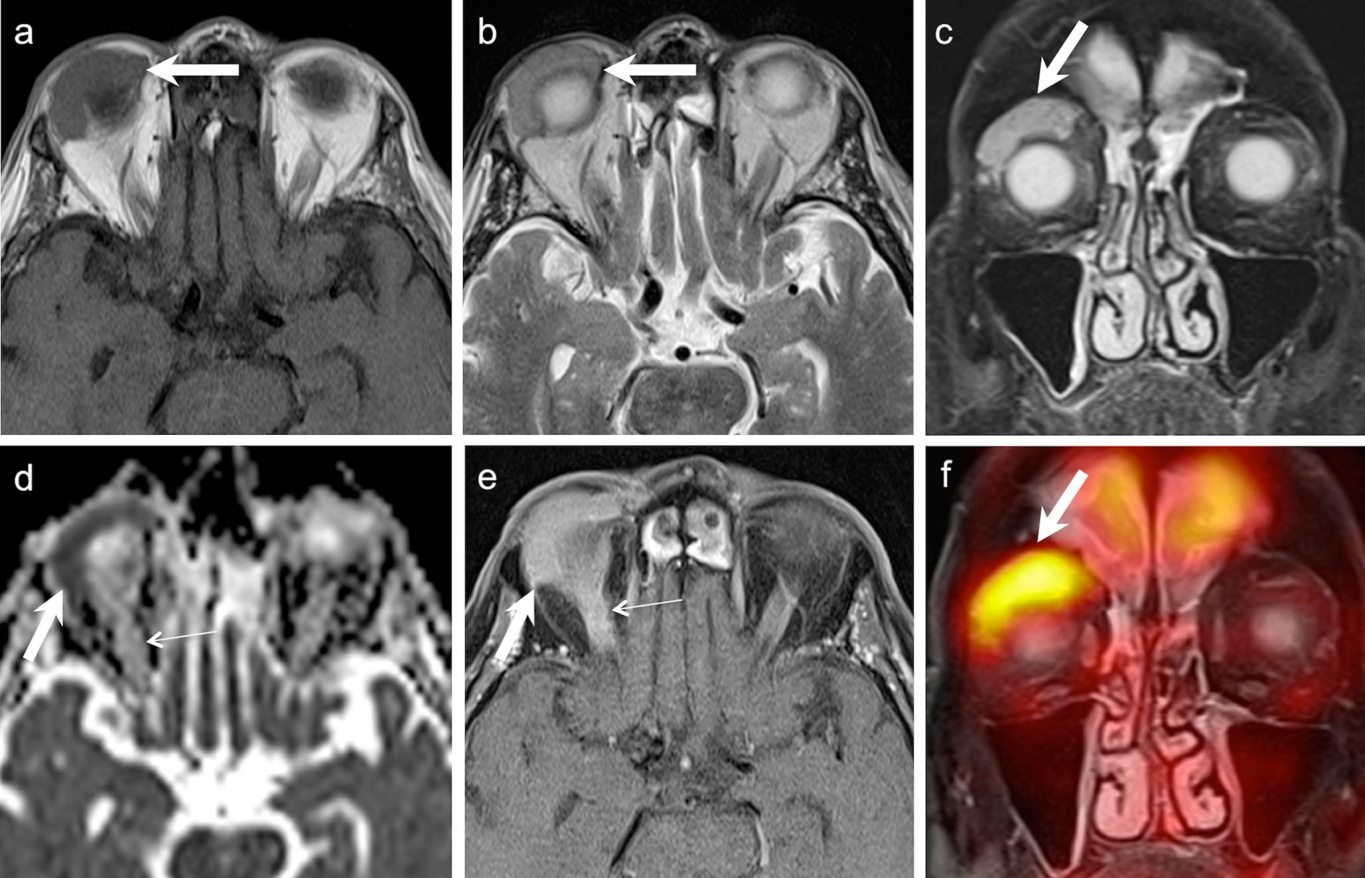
80-year-old male patient with lymphoma of the right orbit. a. Axial T1W image of the orbits shows a hypointense, well-demarcated anterior conal-extraconal lesion (arrow). Axial T2W (b) and coronal STIR (c) images demonstrate intermediate signal intensity of the bulky mass (arrows). Note homogenous aspect. ADC map (d) reveals restricted diffusion (arrow) with very low ADC values (ADC = 0.6 × 10 ^−3^ mm^2^/s) characteristic of lymphoma. Axial contrast enhanced T1W image (e) shows homogenous enhancement of the mass (arrow). There is enhancement of the superior rectus muscle (thin arrow) suggesting possible involvement. However, the ADC map clearly shows that the superior rectus muscle has no restricted diffusion (thin arrows in d and e). f. Coronal fused PET and STIR image from FDG PET/MRI reveals high tracer uptake within the mass (SUVmean = 4.8, SUVmax = 6.7). No other lymphoma manifestations were detected in the body
